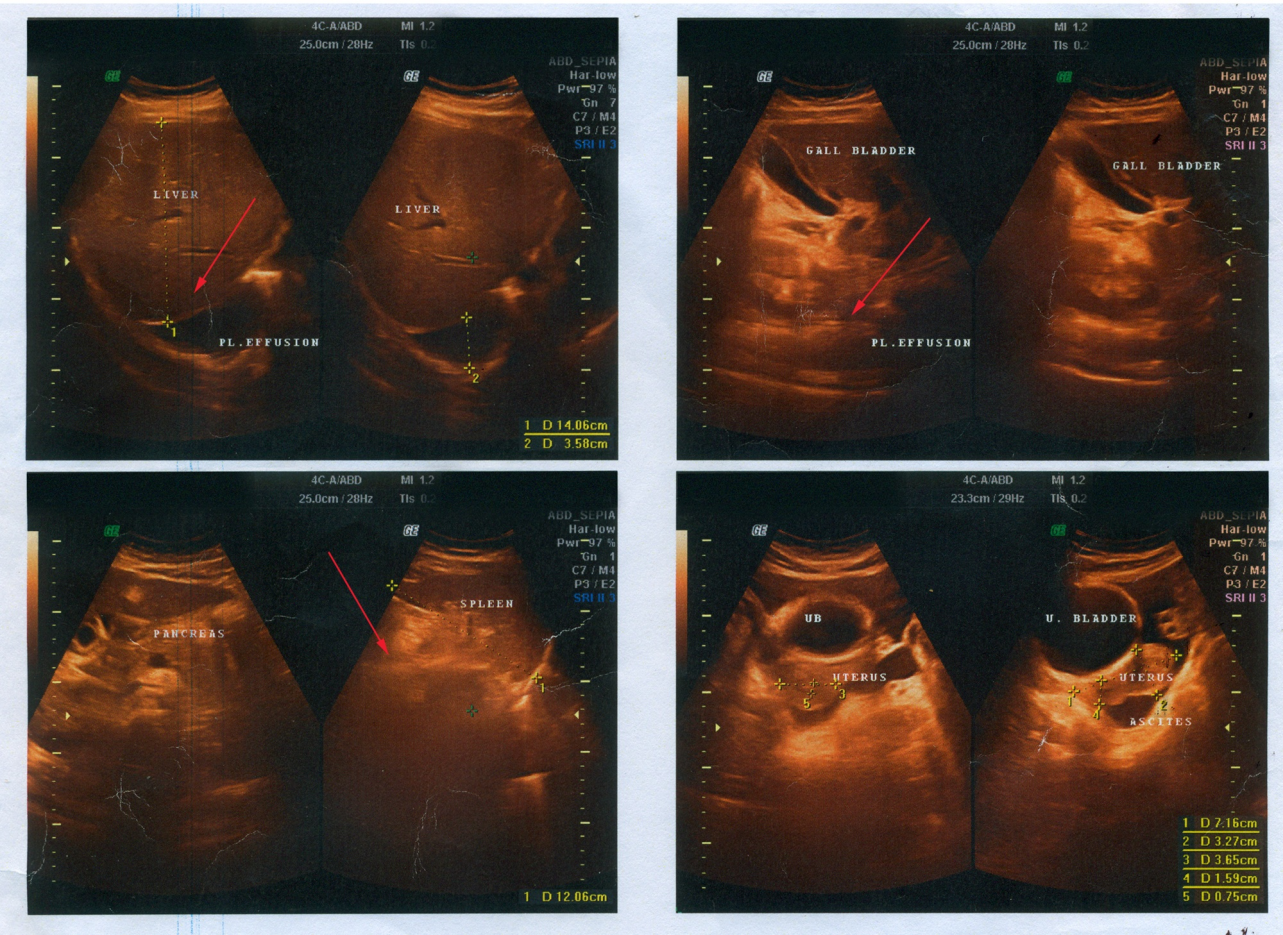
Ultrasonogram of the whole abdomen during hospitalization at day four Mild hepatomegaly with fatty infiltration of the liver (top-left), splenomegaly (bottom-left), pleural effusion and abdominal ascites (top-left and top-right) marked by red arrows.
